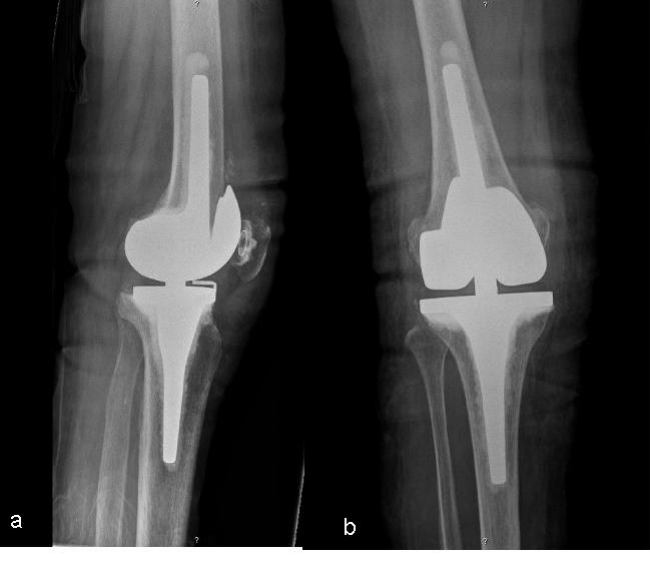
X-ray showing the axial-guided revision TKA (Endoplus®) on a lateral view (a) and a.p. view (b).
